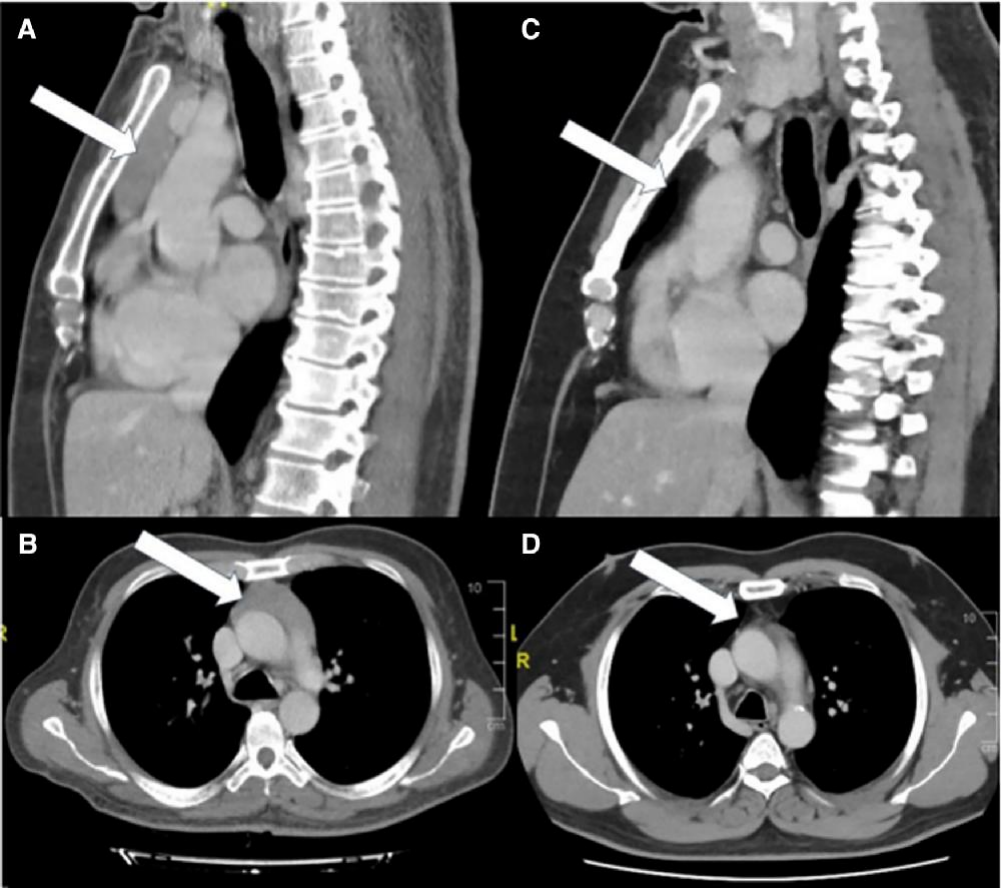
A and B, Initial computed tomography (CT) image at the time of diagnosis showing the enlarged thymus. A, Lateral and B, axial plane views. C and D, CT image after the patient achieved euthyroidism. C, Lateral and D, axial plane views.
